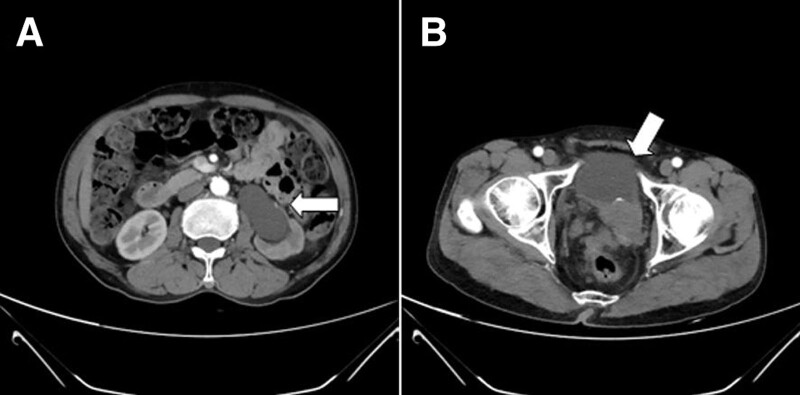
Contrast-enhanced computed tomography (CT) image of the patient with pelvic tumor. (A) Arterial phase scan showing proximal ureter and dilated left hydronephrosis. (B) The white arrow indicates soft tissue mass behind the left bladder. The lesion had invaded the posterior wall of the bladder, and was poorly demarcated from the adjacent bowel.
